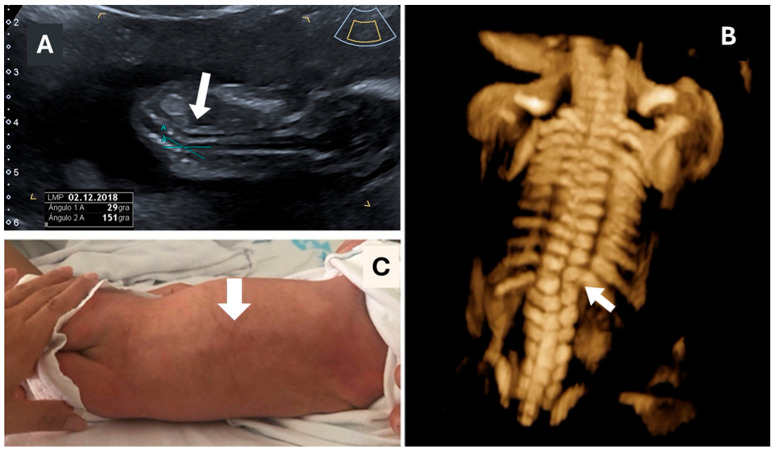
Evolution of a fetus diagnosed with a single hemivertebra (arrows) at the 12-week ultrasound. (A) Coronal view of the fetal spine showing distortion of the dorsal spine at 12 weeks. (B) Three-dimensional ultrasound at 16 weeks revealing the hemivertebra in the dorsal spine. (C) Postnatal images showing the dorsal defect and a change in the spine’s angle. This case was associated with Marfan syndrome, and maternal Marfan syndrome was diagnosed following the fetal diagnosis.
